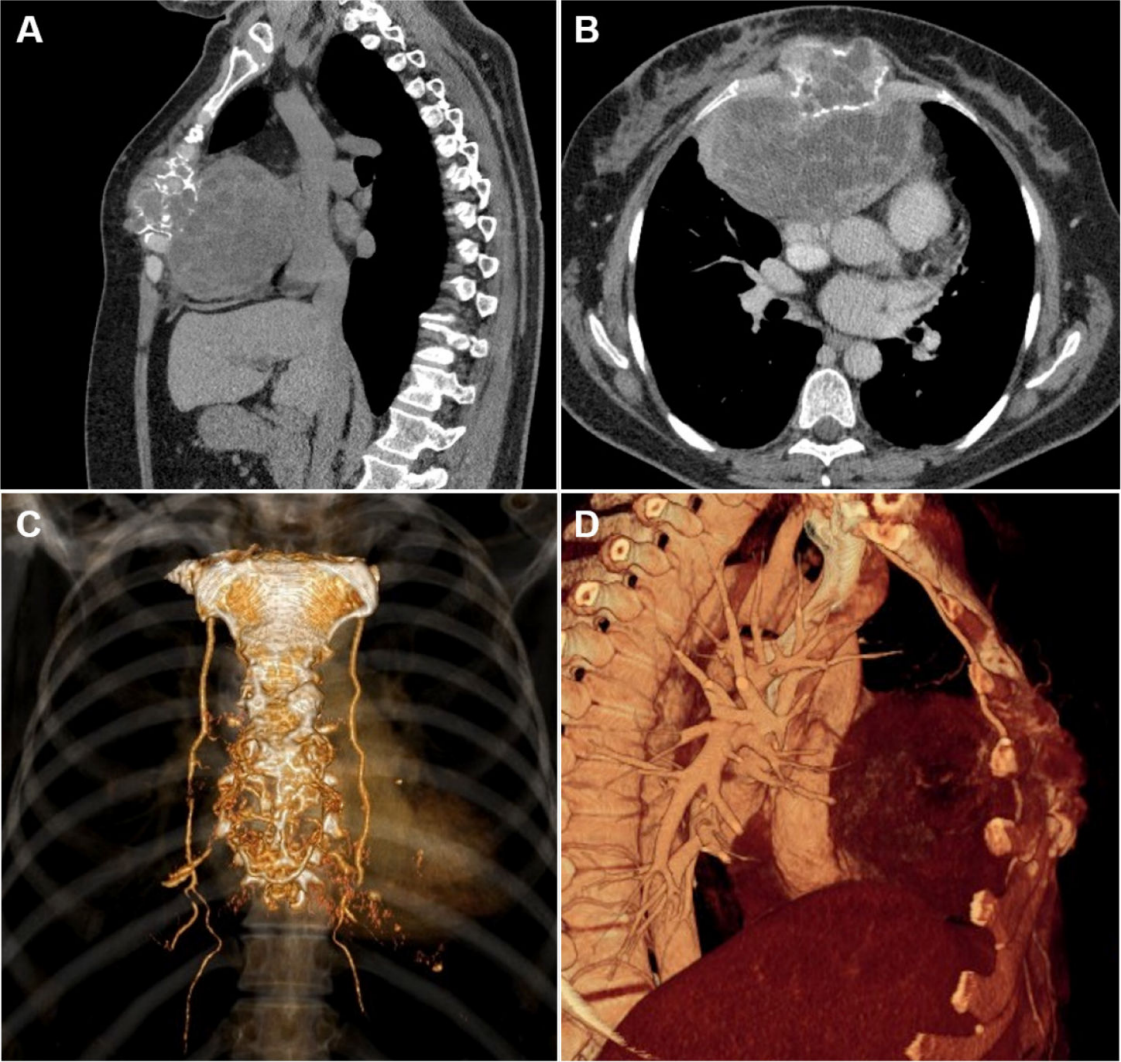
(A) Sagittal and (B) axial chest CT images with (C, D) 3-D reconstruction showing large soft-tissue, osteolytic, heterogeneous sternal mass 12.4cmx9.7cmx8.8cm, with direct extension to the anterior mediastinum, supplied by bilateral internal mammary arteries.
